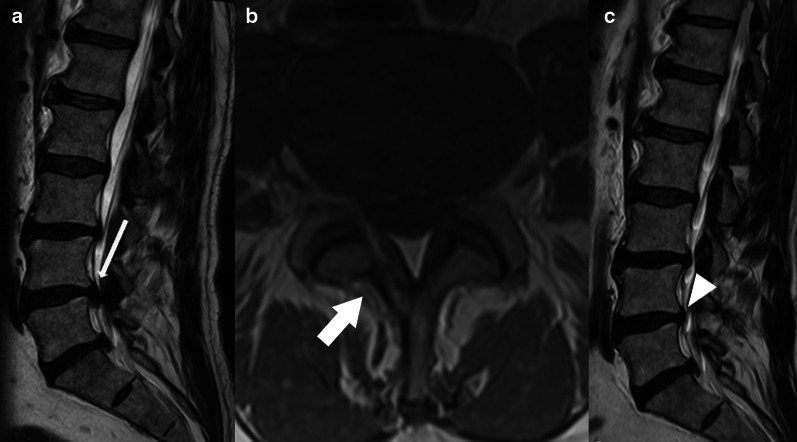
Discectomy and laminotomy. Preoperative T2W sagittal MR image (a) shows the herniated and inferiorly migrated disc at the L4-5 level, abutting the anterior thecal sac (thin arrow) and right L5 nerve root (not shown). There is also a thickened ligamentum flavum. Postsurgical T1W axial (b) and T2W sagittal MR images (c) at the same level as “(a)” demonstrates right laminotomy (arrow) with removal of herniated disc material—discectomy (arrowhead)
